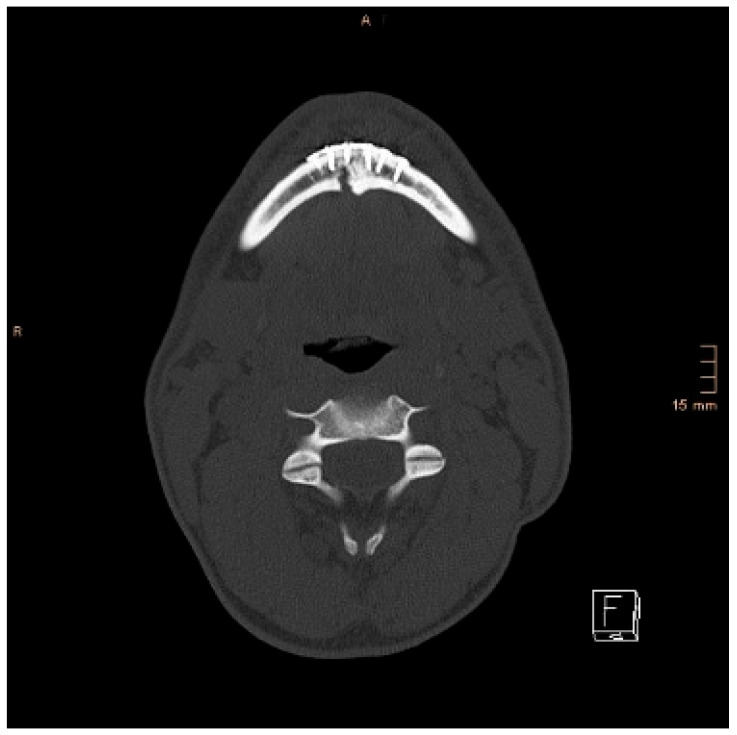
Axial CT scan with splaying of the lingual cortical bone in a symphyseal mandibular fracture after treatment with miniplates.
